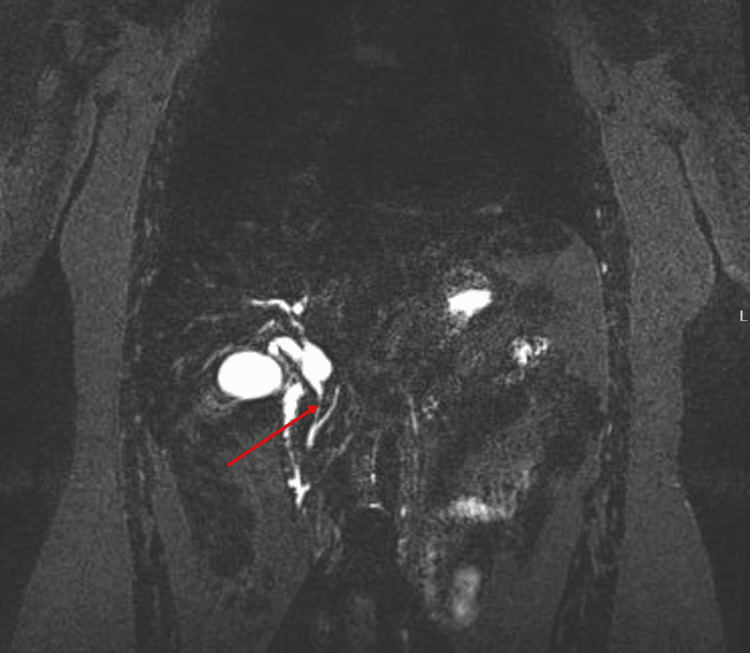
Magnetic resonance cholangiopancreatography (MRCP) T2-weighted image showing common bile duct stricture (red arrow)
